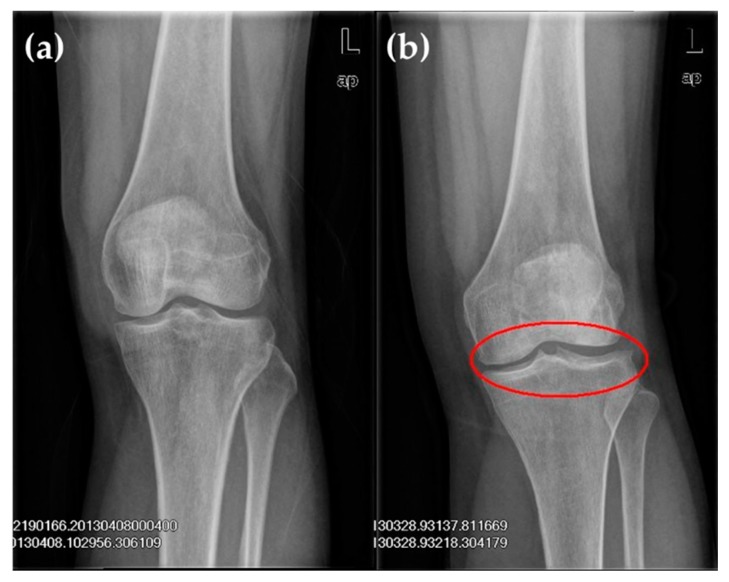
X-ray film of left knee joint: (a) The normal knee showed no obvious abnormal change in the sclerotin of bones in the joint, a well-proportioned joint gap and no obvious swelling of surrounding soft tissue; (b) The knee osteoarthritis (KOA) knee showed protruded left tibial condyles; labial hyperplasia in the external condyle of the tibia, margin of the lateral condyle of the femur and posterior margin of the patella; and a well-proportioned joint gap.
